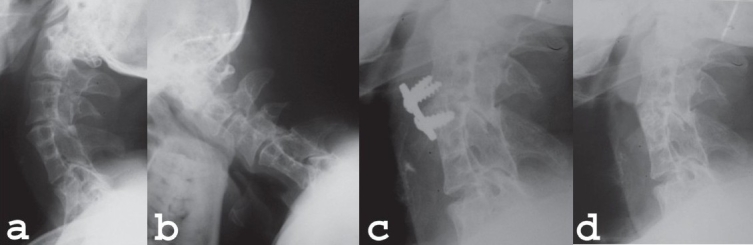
Lateral x-rays of cervical spine (a) showing C3-4 and C5-7 vertebral body fusion with waist formation. The lower surface of C4 is concave and upper surface of C5 in convex. Lateral X-ray cervical spine (b) in flexion shows movements of C4 over C5 vertebral body. (c) Postoperative lateral radiograph of the same patient shows C4-5 interbody plates and screws fixation with screw pulling out. (d) Follow-up X-ray after implant removal shows evidence of sound body fusion
